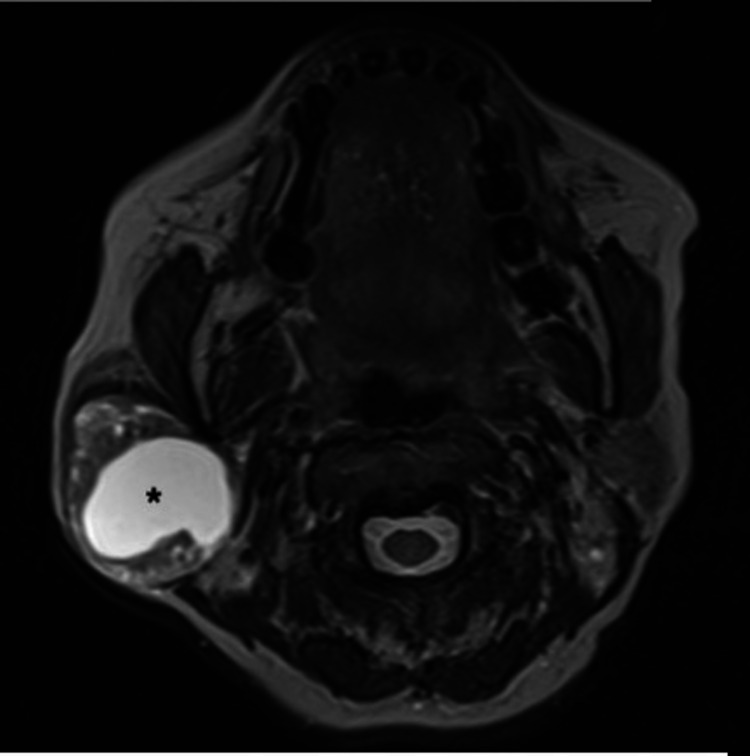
T2-weighted MRI (T2WI). Image showing a well-defined lesion in the right parotid gland with a central homogeneous hyperintense component (*) suggestive of a cyst. Note the heterogeneous peripheral solid component with a few smaller microcysts within.
